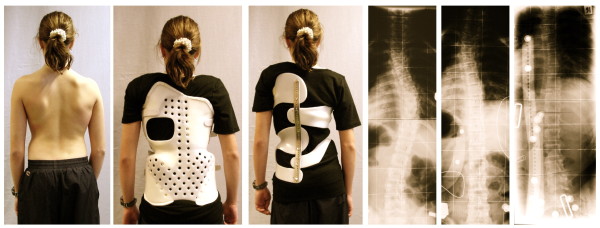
13-year old girl with AIS (39° thoracic). In the previous brace she had 22° high thoracic, 12° low thoracic and 5° lumbar, while in the Chêneau light^® ^brace she has 22° high thoracic, 8° low thoracic and 11° lumbar. The lumbar correction has not been improved after this x-ray in order to achieve a better balance of curves after treatment and a better cosmetic result. The reduction of material in the Chêneau light^® ^brace compared to the previous brace is clearly visible. Brace change was necessary due to severe pains in the previous brace [2].
